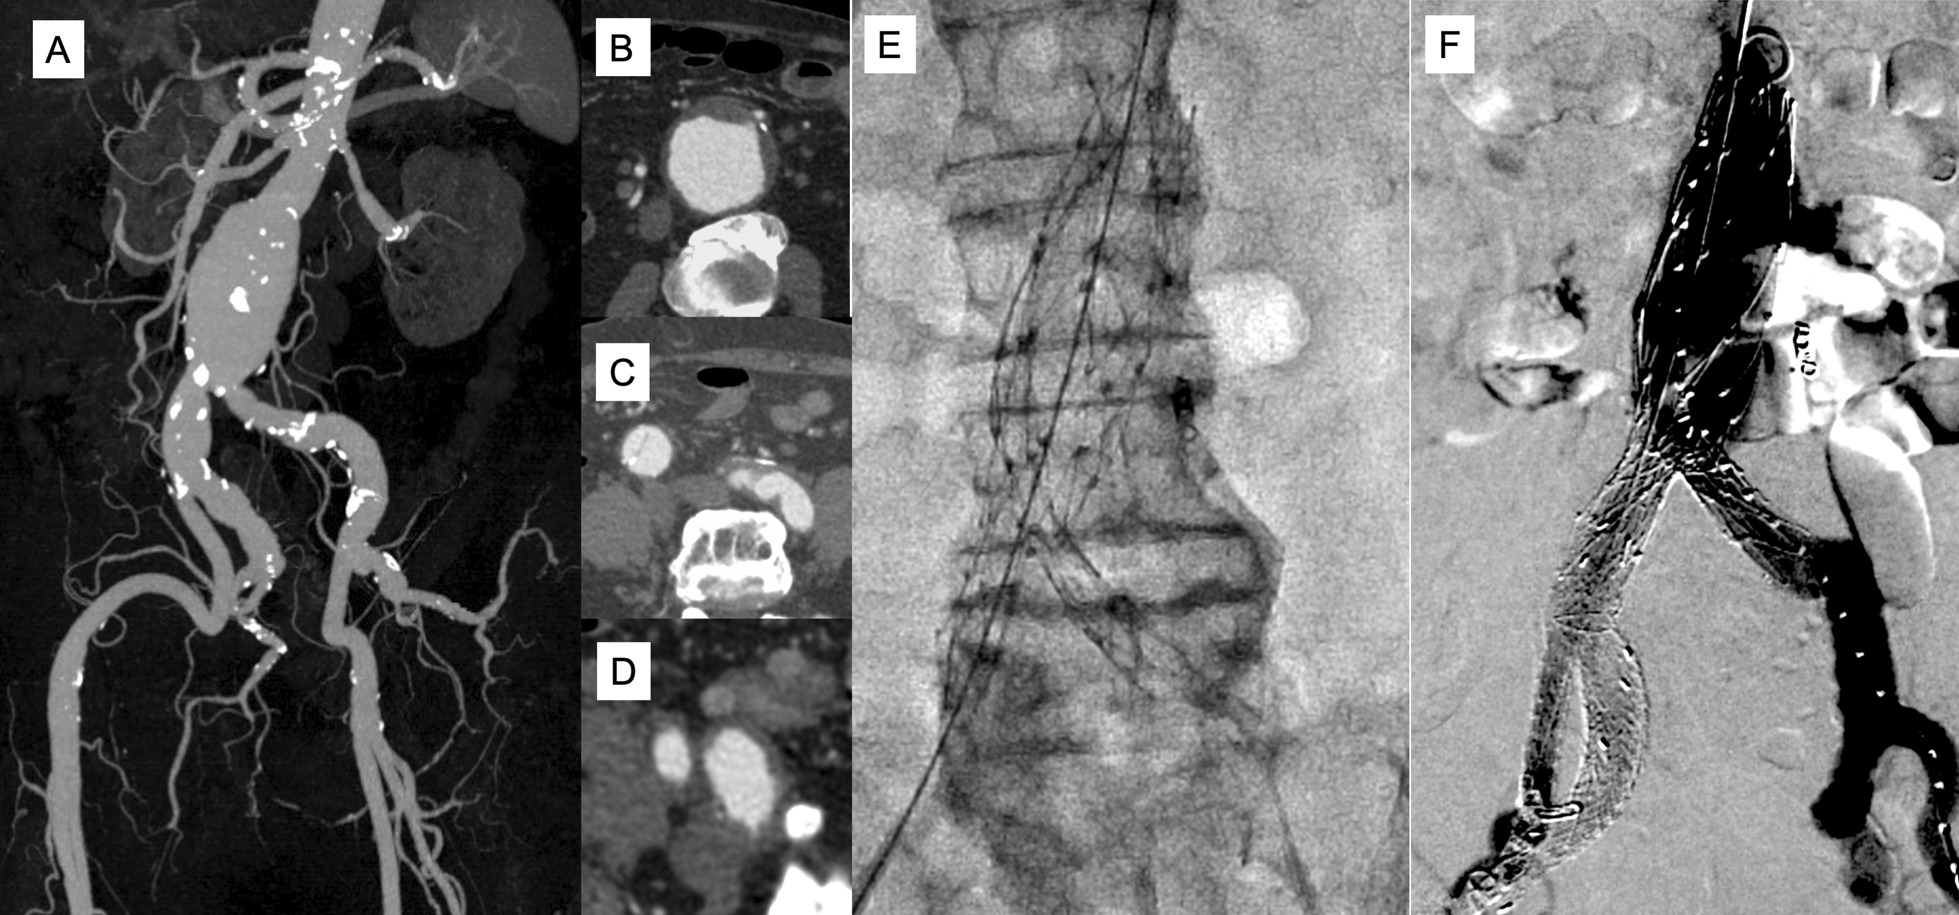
Case 2: A–D Preoperative computed tomography (CT) imaging. A CT angiography (CTA) imaging. The beginning of the right CIA was segmentally stenotic, measuring 13 mm in diameter. B Abdominal aortic aneurysm (55 mm). C The right common iliac artery showing dissecting aneurysm (20 mm). D The proximal portion of right hypogastric artery aneurysm (22 mm). E–F Intraoperative angiogram. E Fluorescent image after placement of AFX2 bifurcated main body. F Completion angiogram showing proper blood flow to right external and inferior gluteal artery without any endoleaks
